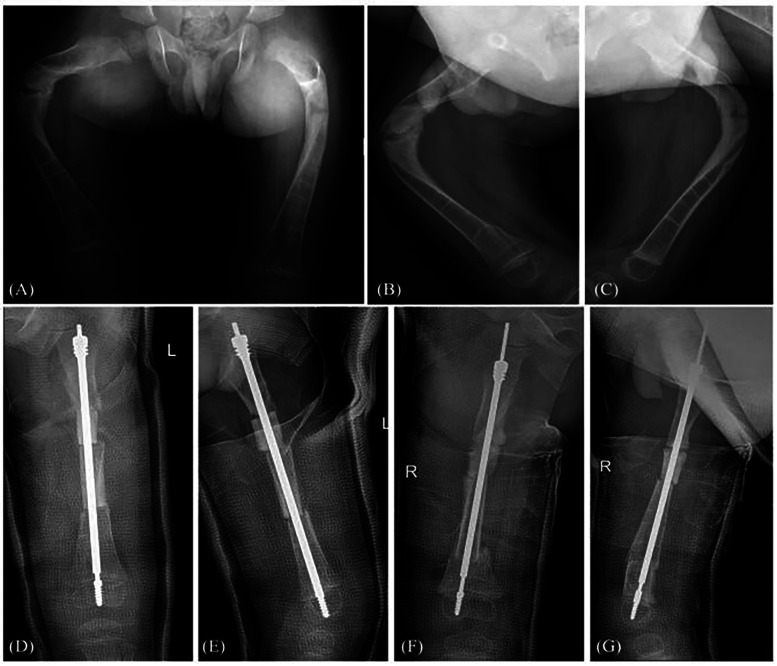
Radiographs of the bilateral femurs in a 3-year-old patient. (A–C) Show anteroposterior and lateral views of bilateral femoral fractures and deformity. (D,E) Show immediate postoperative anteroposterior and lateral views of the left femur after FD nail internal fixation and osteotomy correction. (F,G) Show immediate postoperative anteroposterior and lateral views of the right femur after FD nail internal fixation and osteotomy correction.
